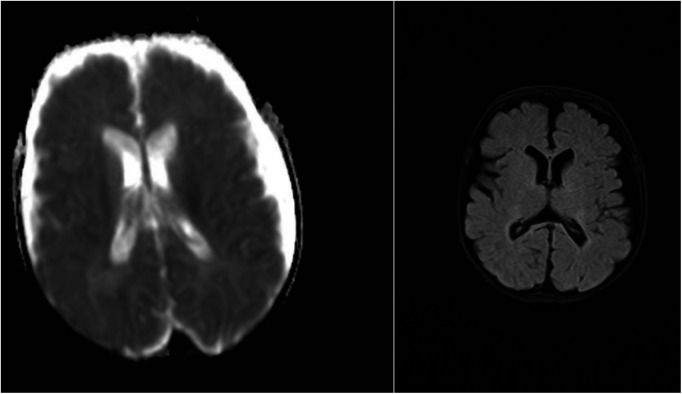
MRI screening with no abnormal anatomic structure in a three-year-old male patient with NDD.
